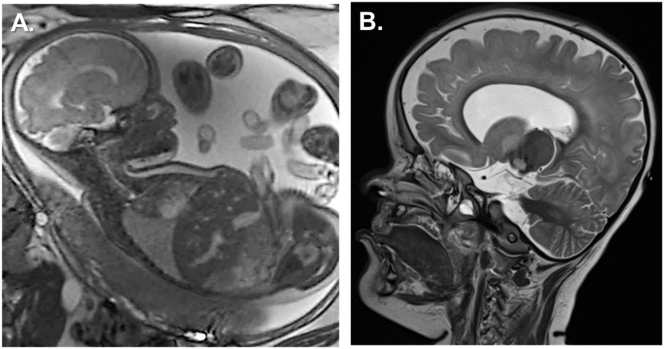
Fetal and cerebral magnetic resonance imaging (MRI). (A) Fetal MRI at 32 weeks showed: flat orbital roof, suspected hypoplastic orbital conus, hypoplastic bulb on both sides, shortened nasal septum, flat nose and bilateral cleft lip. Anophthalmia and microphthalmia were undifferentiated. (B) T2-weighted MRI of the central nervous system at age two showed: brain parenchymal hypoplasia, extensive diffusion restriction to the mesencephalon, bilateral anophthalmia with aplasia of the anterior visual pathway, non-developed pituitary gland causing panhypopituitarism.
